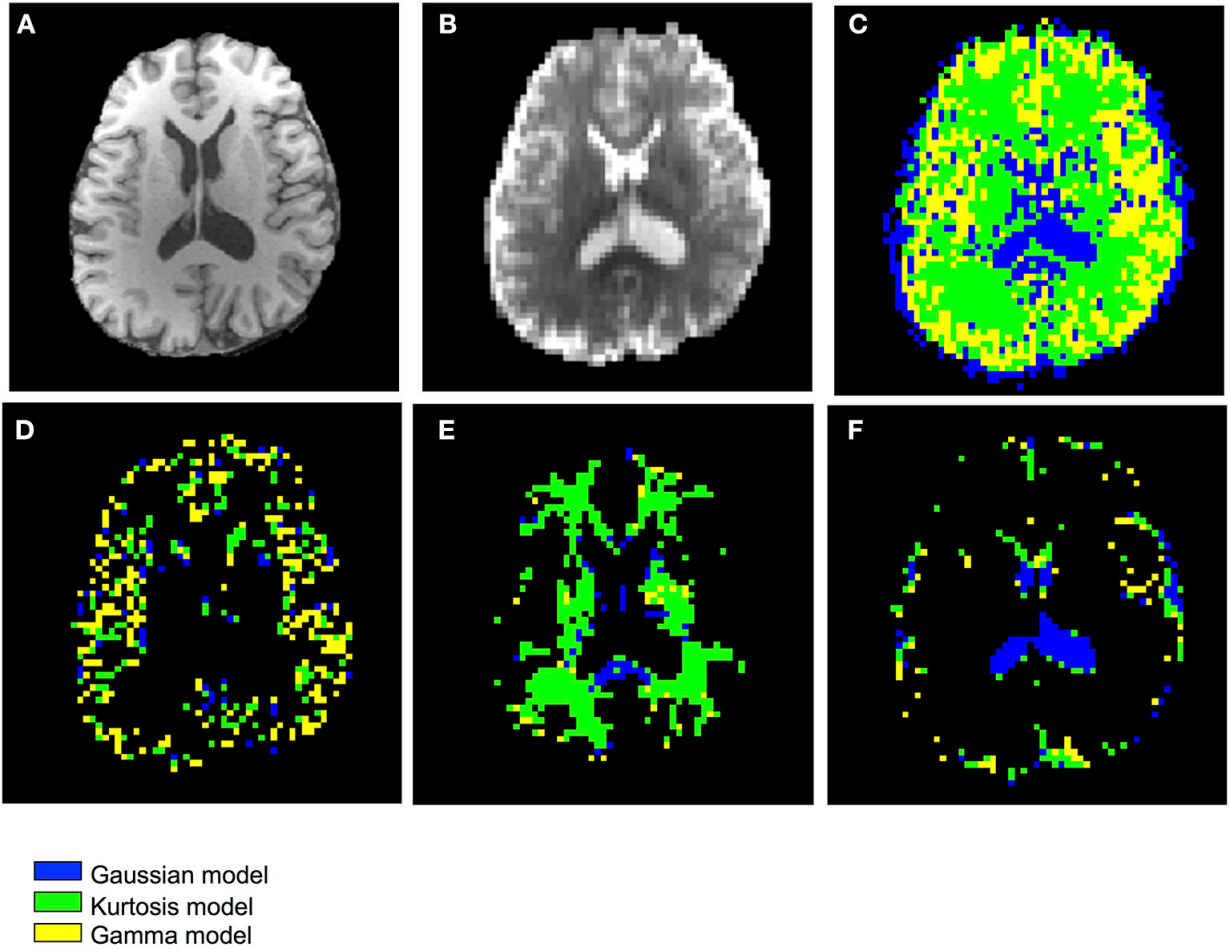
Demonstration of the anatomical maps and the optimal model maps: (A) T1-weighted (MP-RAGE) image (B) b_0_ image of the IVIM-MRI series (C) optimal model map (D) optimal model map of 95% GM (E) optimal model map of 95% WM (F) optimal model map of 95% CSF.
